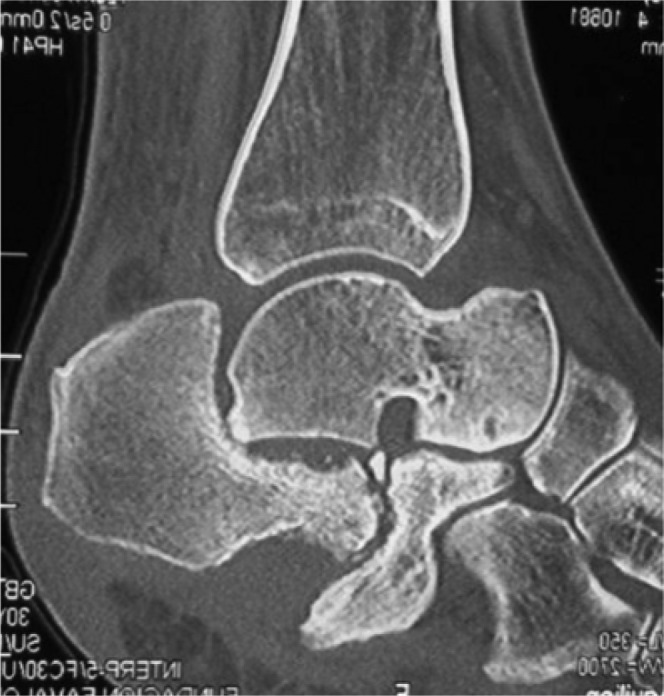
Sagittal view of a computed tomography scan showing pseudoarthrosis and remodelling of the calcaneal tuberosity.
